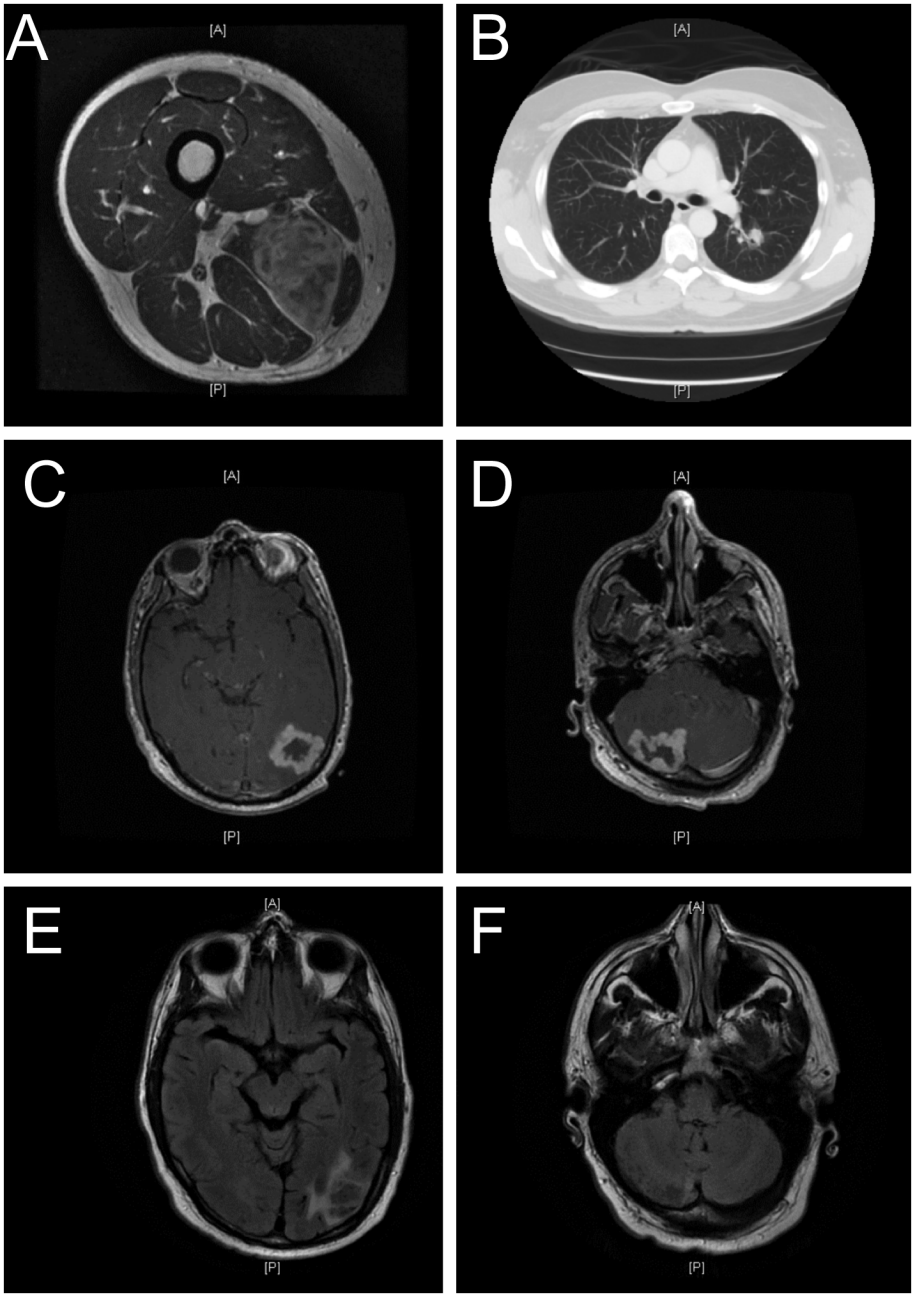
Imaging from diagnosis through recovery depicting the clinical course of *C. gattii* infection. A) MRI imaging of the upper thigh cryptococcoma. B) CT imaging of a pulmonary nodule, likely to be a cryptococcal granuloma. C–D) MRI imaging of brain cryptococcomas after seizure presentation at the emergency room. E–F) MRI imaging of brain cryptococcomas after long-term fluconazole treatment, with reduced mass.
